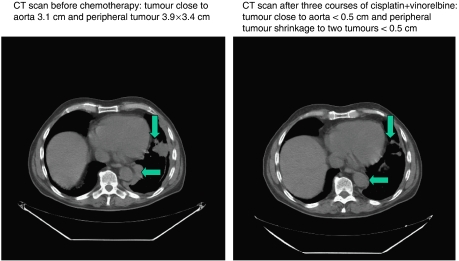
Same patients as Figure 1 with CT scan before and after three courses of cisplatin and vinorelbine in MPM with shrinkage of tumours more distally close to aorta and peripheral tumour.
